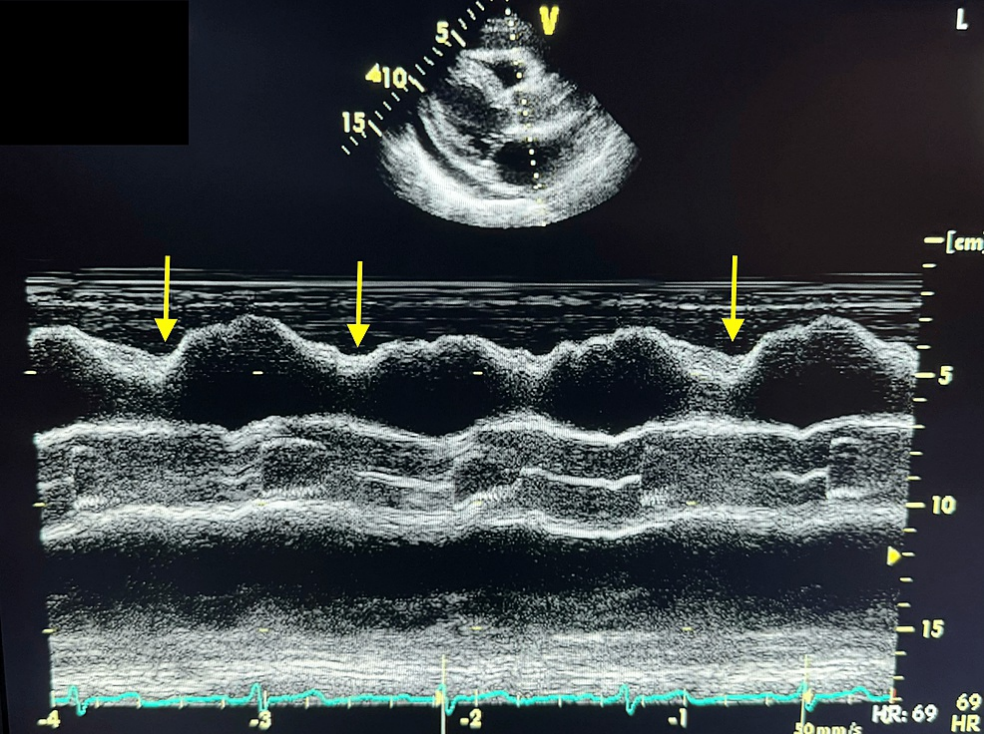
M-mode TTE from parasternal long-axis view showing evidence of right ventricular collapse during early diastole The yellow arrows denote the regions of right ventricular collapse during diastole. M-mode: motion mode; TTE: transthoracic echocardiogram
